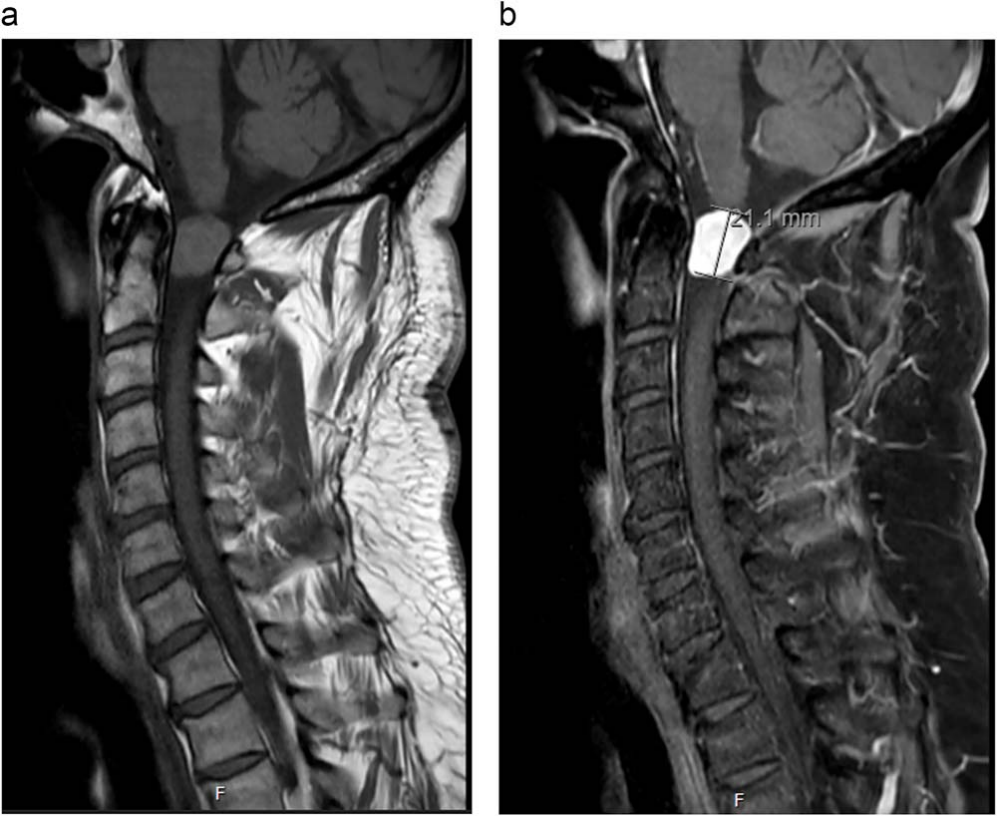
(a) Pre-operative sagittal MRI cervical spine T1-weighted image without contrast. (b) Pre-operative sagittal MRI cervical spine T1-weighted image with contrast.
